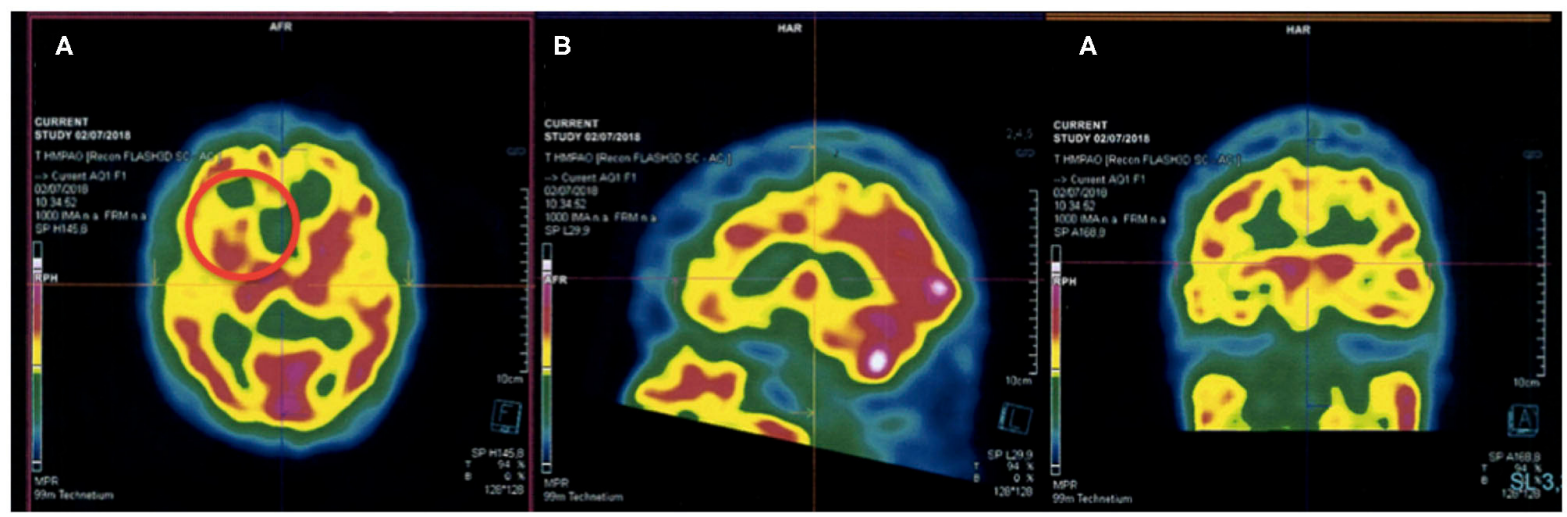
Brain perfusion SPECT showing (A) hypometabolism of the right dorsal striatum (red circle), (B) hypometabolism of the frontal lobes, and (C) hypometabolism of parieto-occipital lobes.
